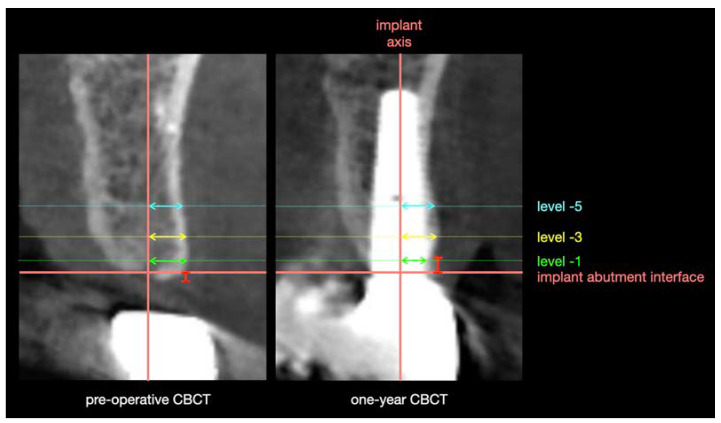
The following reference lines were constructed on the one-year CBCT in the center of the implant: the long axis of the implant, the level of the implant-abutment interface perpendicular to the long axis of the implant, level −1 mm, level −3 mm and level −5 mm apical to the implant-abutment interface and perpendicular to the long axis of the implant. Horizontal measurements were performed between the center of the implant and the buccal bone surface at the different levels (level −1 mm: green arrow, level −3 mm: yellow arrow, level −5 mm: blue arrow). Vertical measurement was performed between the implant-abutment interface and the buccal bone peak, parallel to the long axis of the implant (red arrow).
